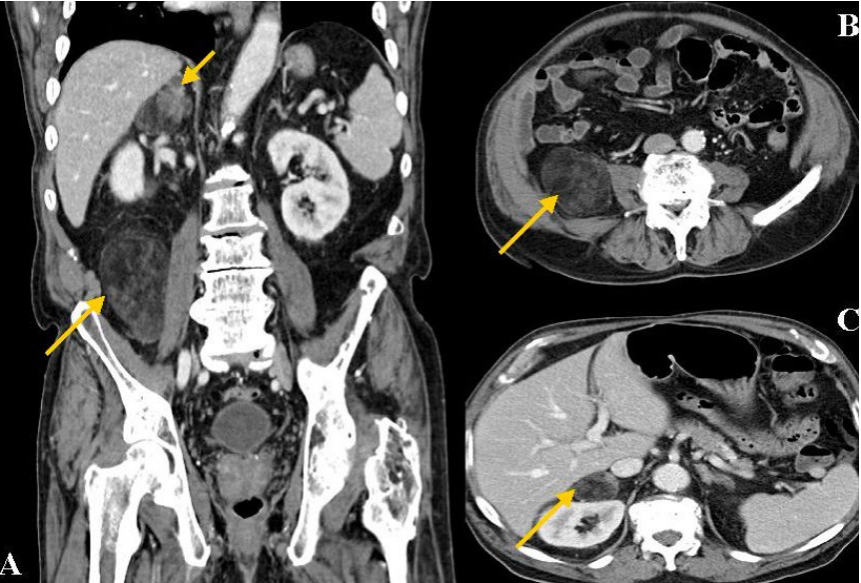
CT imaging of the abdomen. A: Coronal abdominal CT scan detected two fat-containing masses in the adrenal gland and lateral of the psoas muscle inferior to the right kidney. Both lesions appeared identical in the CT imaging and were at first consistent with a well-differentiated liposarcoma. Intra-operative biopsy followed by rapid section revealed a simultaneous adrenal and extra-adrenal myelolipoma. B and C: Further CT images of the extra-adrenal/retorperitoneal myelolipoma.
